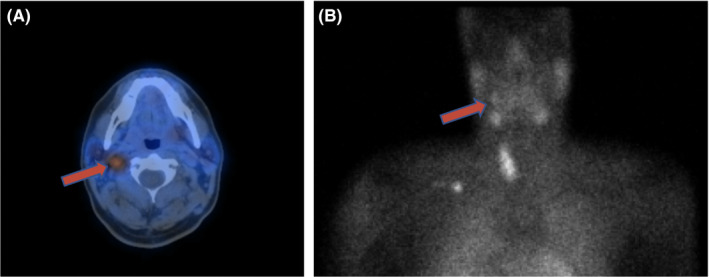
A, Fusion SPECT sestamibi parathyroid scintigraphy demonstrating uptake in the right carotid sheath. B, Anterior global image of sestamibi parathyroid scintigraphy showing focal uptake in the right carotid sheath and physiologic uptake in the bilateral parotid glands, bilateral submandibular glands, and the remaining right thyroid lobe
